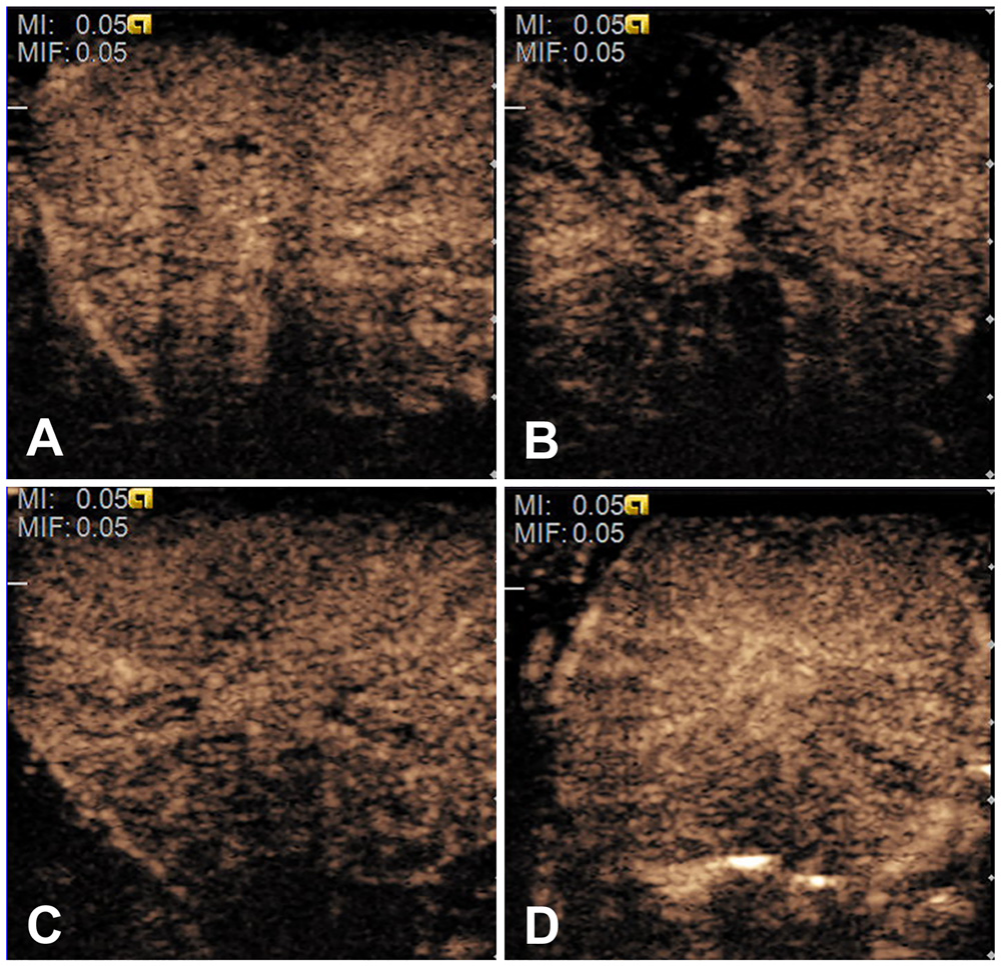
Contrast-enhanced ultrasound images of the microbubble-enhanced ultrasound and prothrombin and prothrombin-treated prostates. (B) The microbubble-enhanced ultrasound and prothrombin-treated prostate, demonstrating a significant blood perfusion decrease in the targeted area. (A, C, D) The baseline in the microbubble-enhanced ultrasound and prothrombin group (A) and all of the corresponding contrast-enhanced ultrasound images (C: before treatment, D: after treatment) of the prothrombin-treated prostates, showing homogenous blood perfusion.
